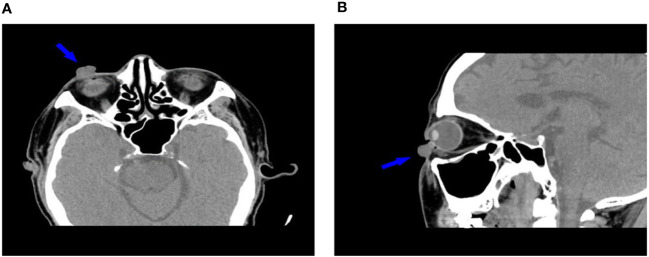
Head computed tomography (A: transverse plane, B: sagittal plane) shows the relationship between the nodule and surrounding soft tissues (arrows).
